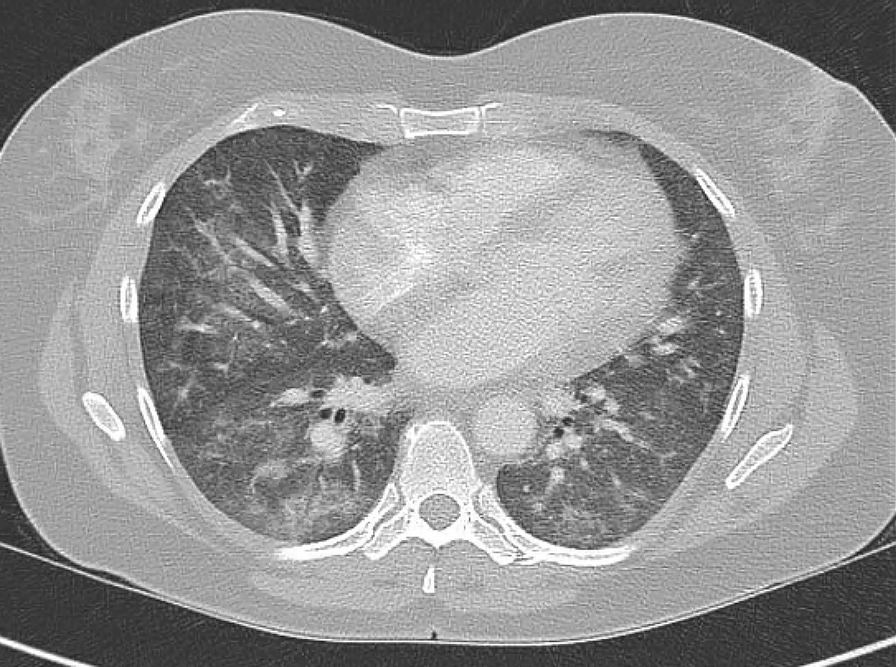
Computed tomography angiography of the chest revealing bilateral ground glass opacities, with no filling defects suggesting pulmonary emboli
